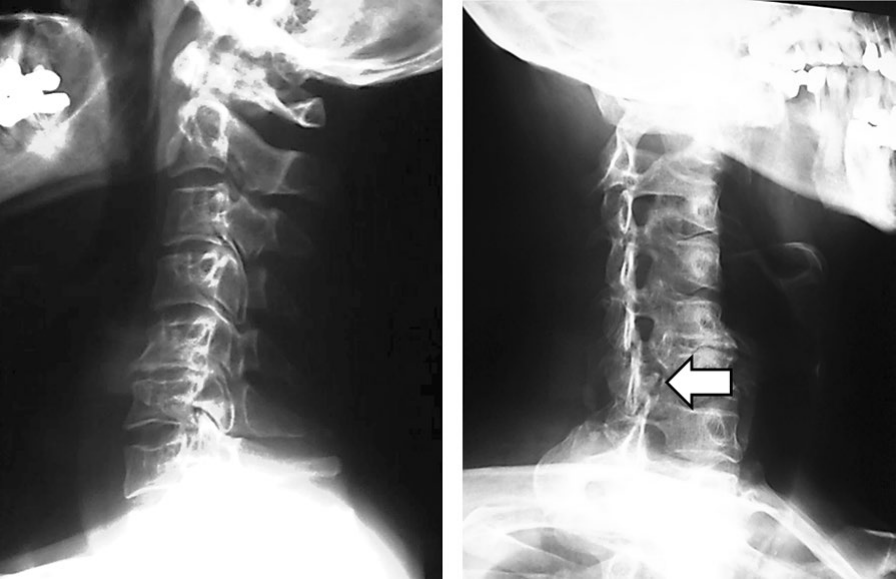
Preoperative plain radiograph of the cervical spine. An osseous protrusion in the right C6/7 foramen could be observed
